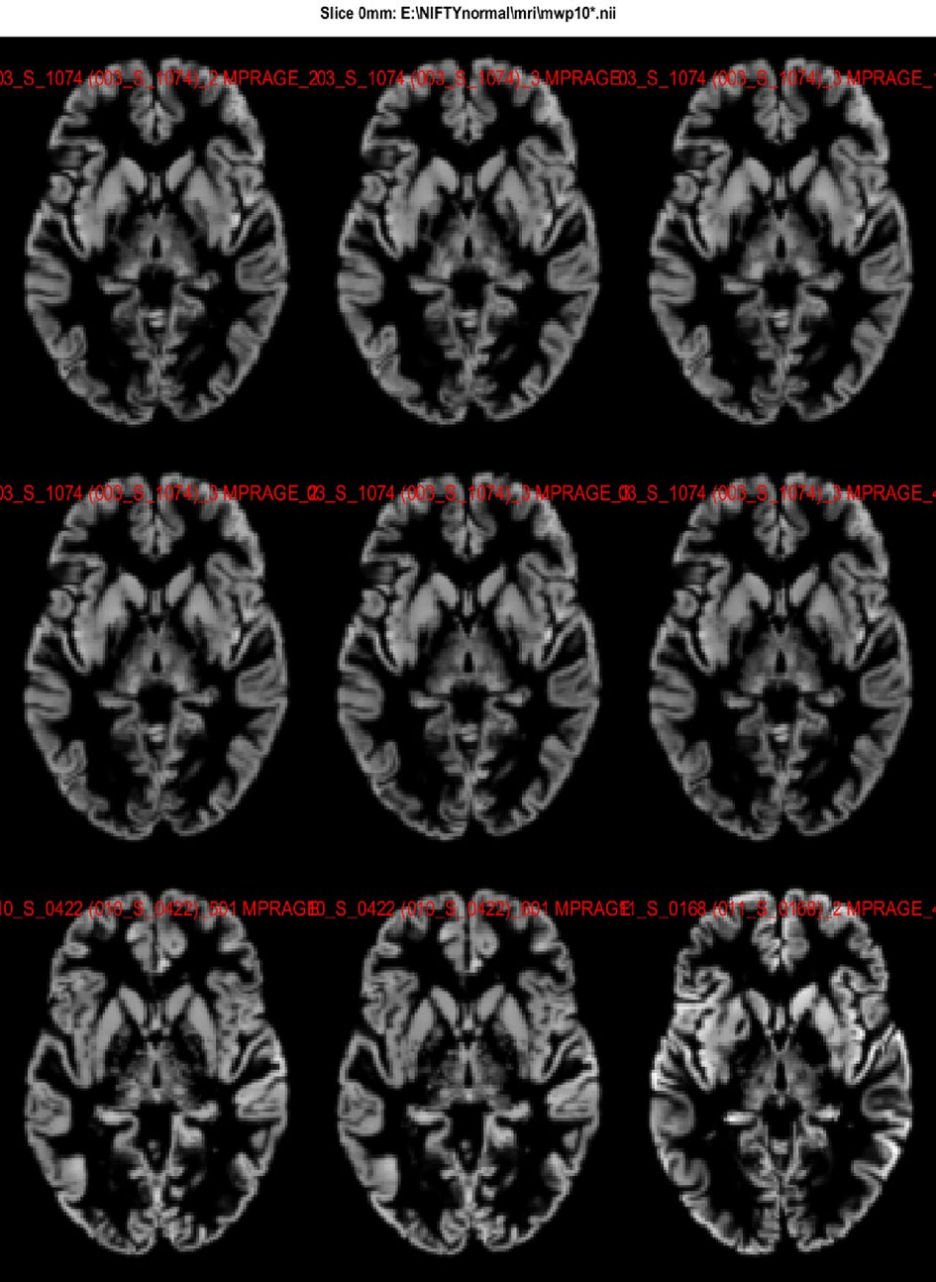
Axial single slices from multiple preprocessed MRI scans used to validate image orientation and spatial normalization across subjects. These slices ensure consistent alignment and anatomical correspondence for accurate downstream analysis.
